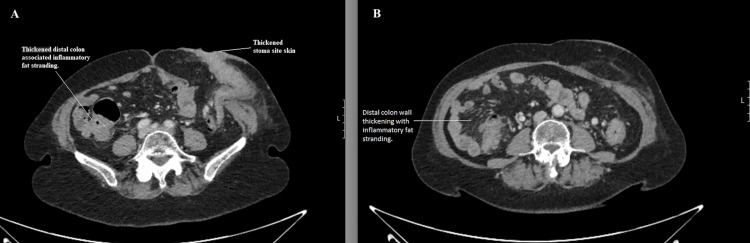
Computed tomography (CT) of the abdomen showing colitis of the distal colon with stomal cellulitis (A) Axial CT section showing colitis with inflammatory fat stranding and thickening at the stoma site; (B) axial CT section showing inflammatory fat stranding
